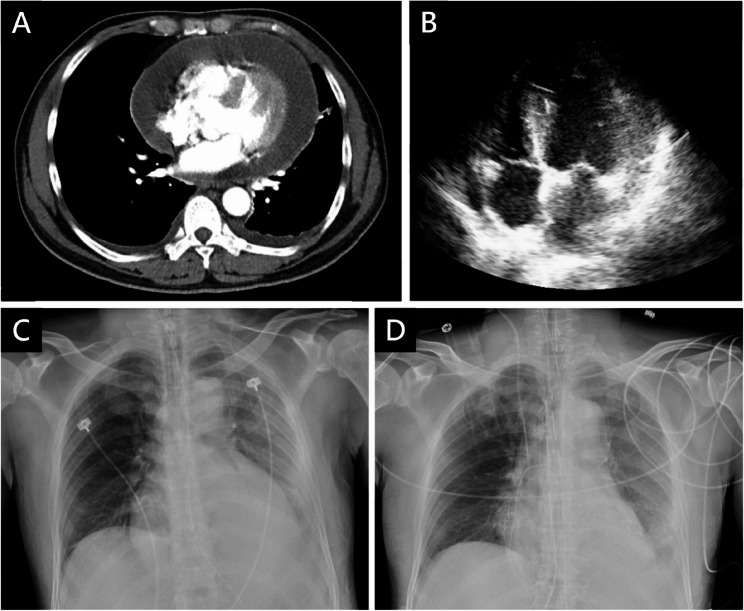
Preoperative Imaging of the Chest Upon Admission and Postoperative Radiograph. (A) Chest CT conducted at the former hospital revealed a 25.79 mm large pericardial effusion surrounding the entire heart. (B) TTE subcostal view showed a 25.48 mm pericardial effusion. (C) CXR (AP view) showed an enlarged heart configuration. (D) CXR (AP view) after partial pericardiectomy demonstrated a smaller heart size
